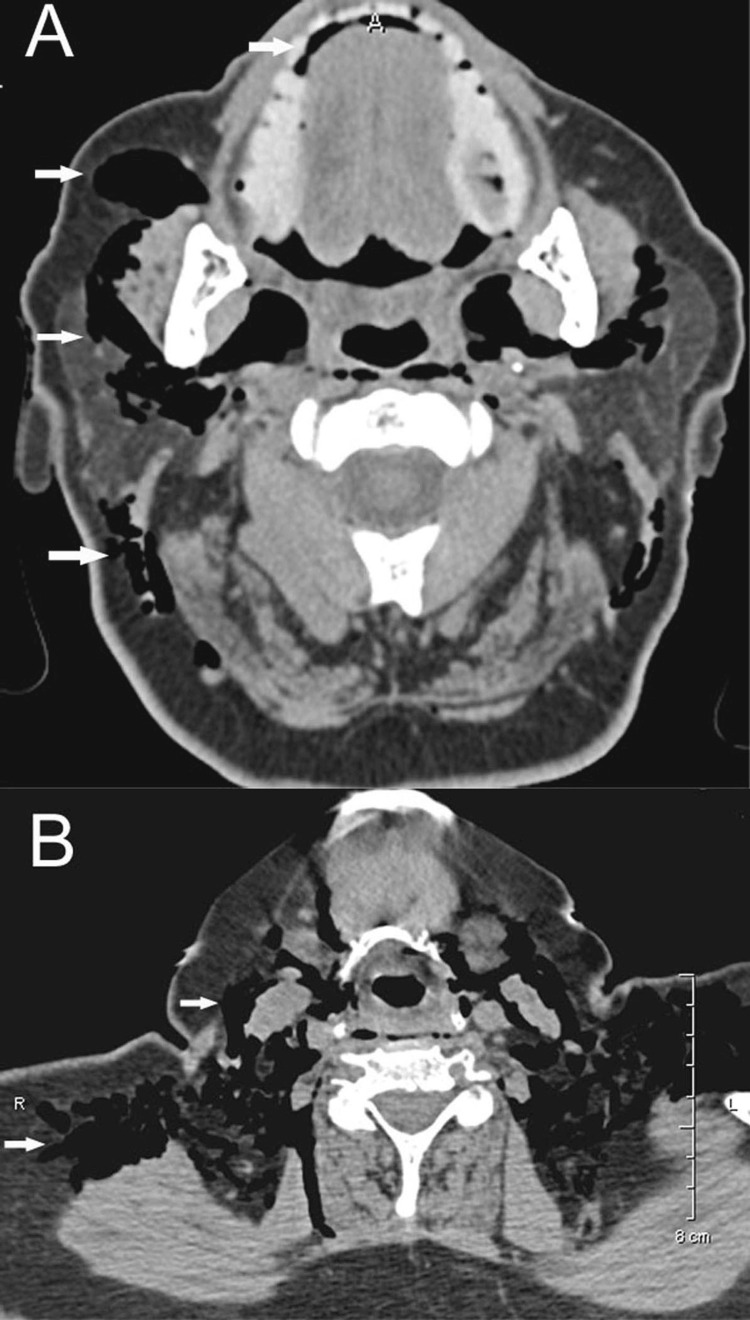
Scans of an 81-year-old woman who committed suicide with a knife, stabbing her face and neck Axial CT images (A) and (B) depict multiple pockets (arrows) of craniocervical and cervicothoracic free air.
